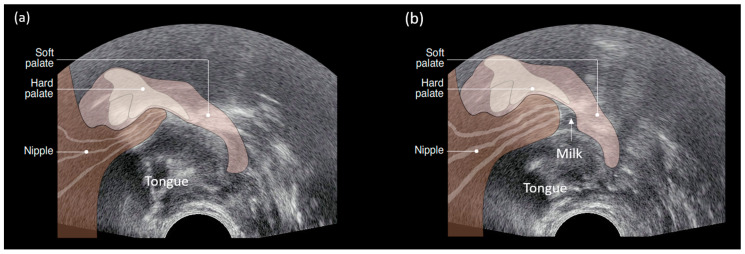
Ultrasound images of the infant oral cavity during breastfeeding (a) Tongue up position during breastfeeding. (b) Tongue down is drawn down to create a vacuum and milk flows into the oral cavity. ©Medela AG 2006. Used with permission.
